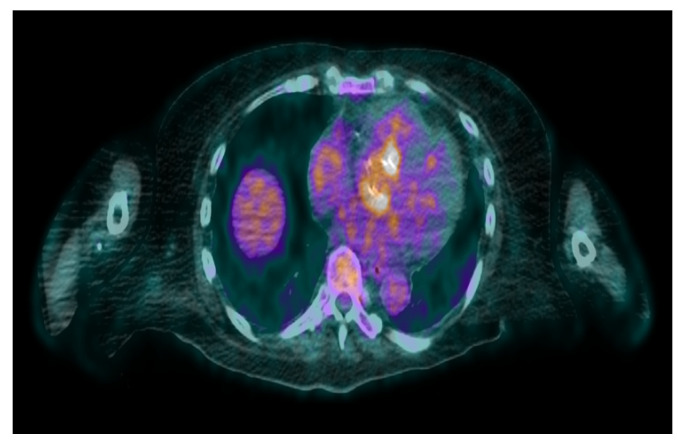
Native aortic valve infection. 18F-FDG PET/CT fused image shows intense uptake of the radiotracer (18F-FDG) localized to the aortic valve of the patient. Diagnosis via transesophageal echocardiography was challenging due to the extensive fibrocalcifications of the aortic valve apparatus in an elderly patient (male, 82 years old).
